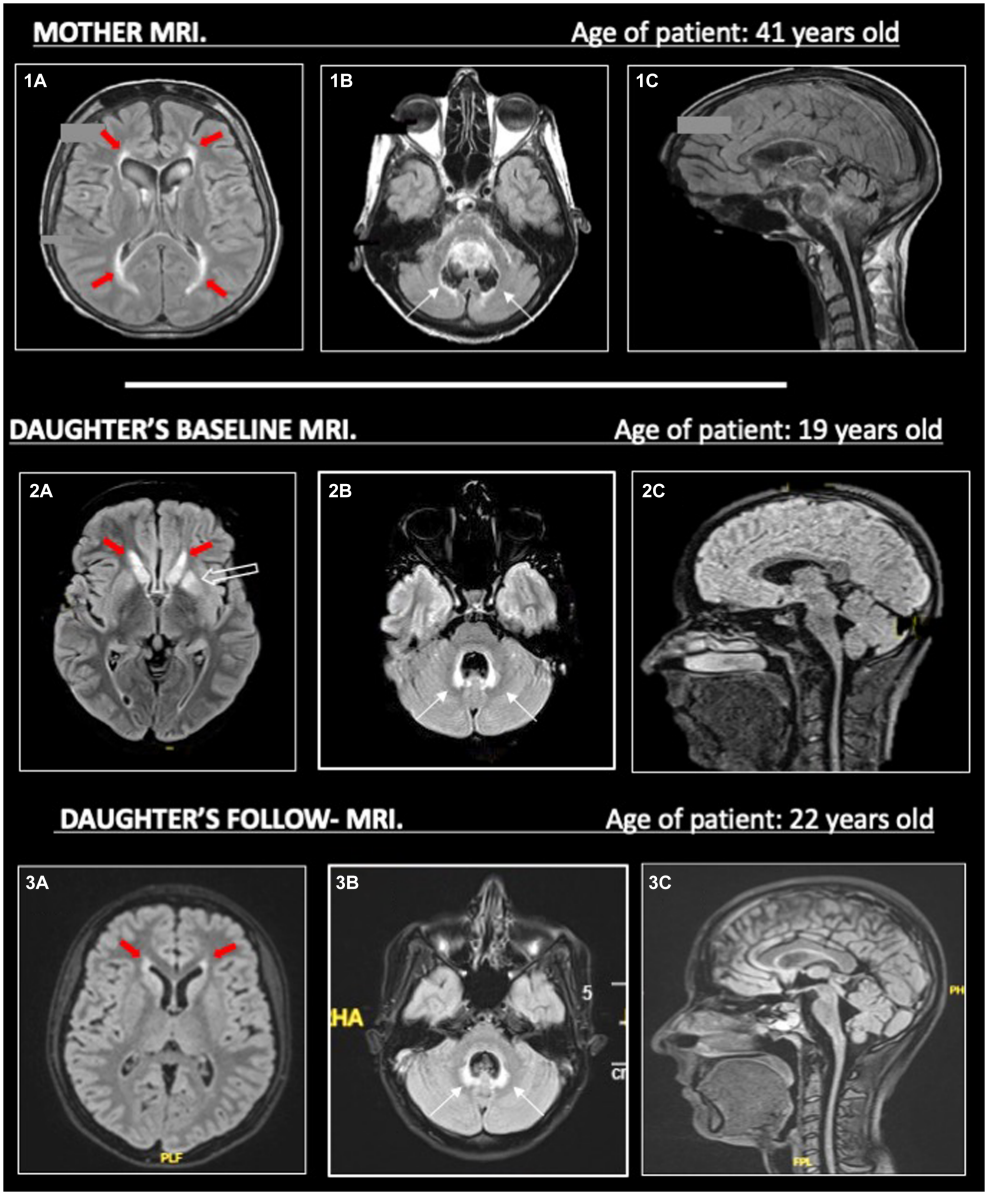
On the left (1a, 2a, 3a), we show T2-hyperintense symmetric periventricular lesions (red-filled arrows) in both mother and daughter. In (2a), we can see T2-hyperintensities in the basal ganglia (empty arrow) of the daughter, less evident in her MRI follow-up in 2023 (3a). On the central column (1b, 2b, 3b), widespread atrophy of the cerebellar vermis is evident in both mother and daughter (1b, 2b), without evident worsening in the follow-up MRI (3b) in the daughter. T2-hyperintensities around the IV ventricle (thin black arrows) are more pronounced in the daughter than the mother, maybe due to a higher cerebellar vermis atrophy. On the right (1c, 2c, 3c), in the sagittal images, we can see prominent atrophy of the cerebellum, brainstem (with spare of pons) and cervical spinal cord. All MRI sequences are FLAIR, apart from (1c) (T1).
